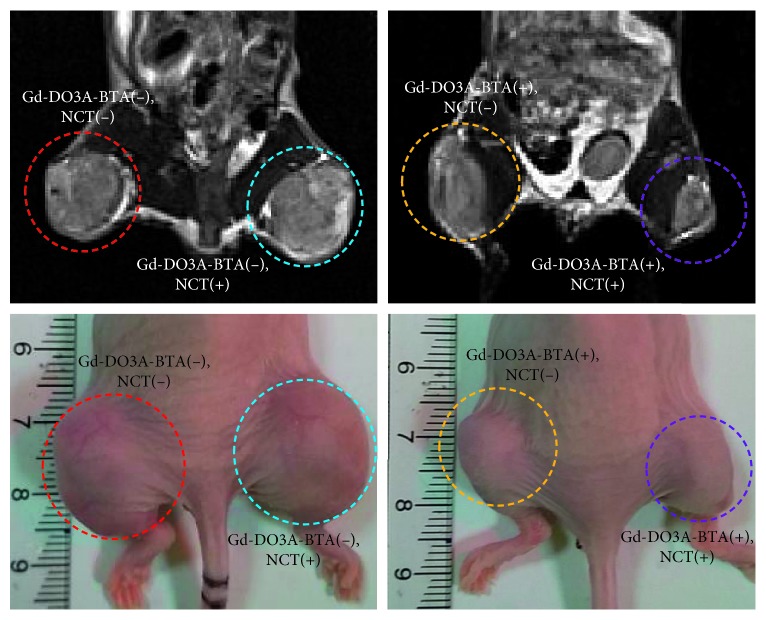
MRI (above) and morphological findings (below) of tumors at 60 days after Gd-NCT. [Gd-DO3A-BTA(+), NCT(+)], Gd-DO3A-BTA administered and neutron irradiated; [Gd-DO3A-BTA(+), NCT(−)], Gd-DO3A-BTA administered and non-neutron irradiated; [Gd-DO3A-BTA(−), NCT(+)], non-Gd administered and neutron irradiated; [Gd-DO3A-BTA(−), NCT(−)], non-Gd administered and non-neutron irradiated.
